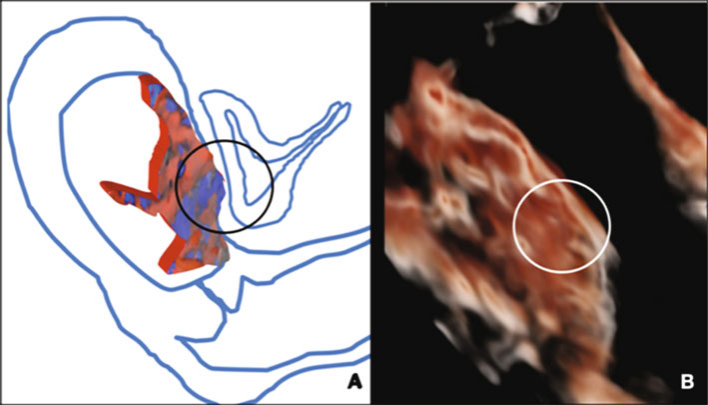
(a, b) Images of utero-bladder relationship. Graphic reconstruction (a) and 3D-ultrasound image (b) of the focal myometrial invasion without involvement of the bladder and integrity of the posterior bladder wall.
